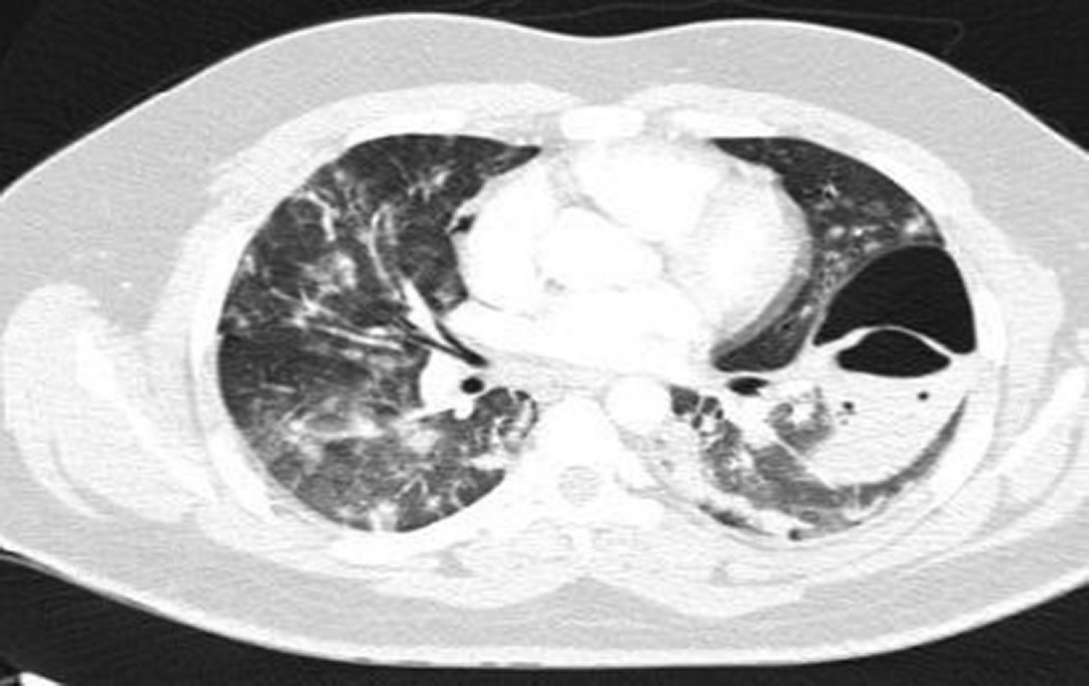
Chest CT scan in the ER on the second day of the presentation
